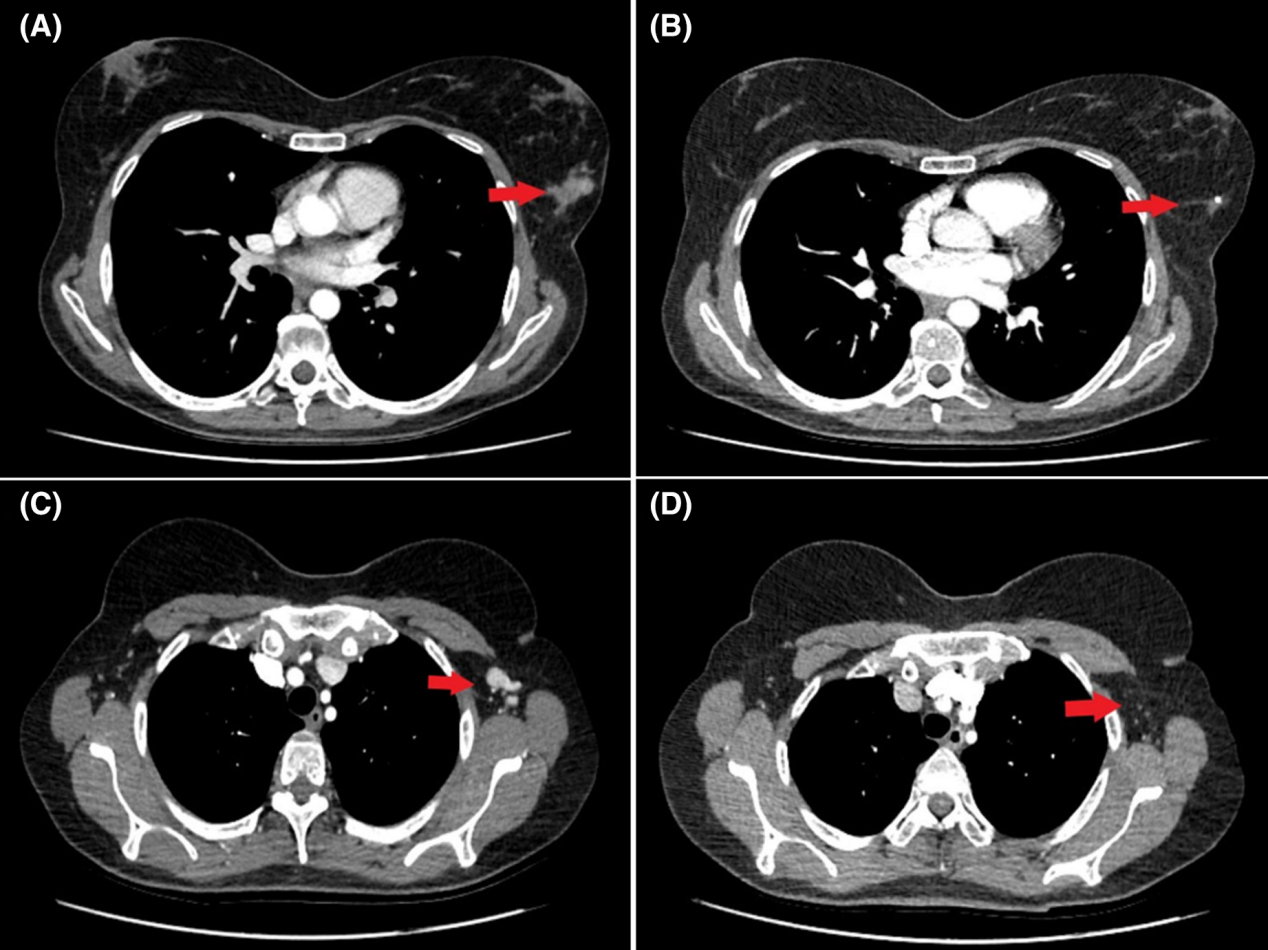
Contrast‐enhanced computed tomography scans of the thorax. Left breast mass before treatment initiation (A) and after 3 months of therapy (B). Left axillary lymphadenopathy before treatment initiation (C) and 3 months after (D). Red arrows indicate the tumor area.
